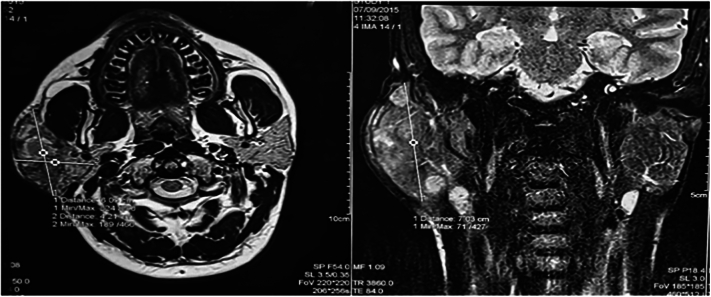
MRI showing axial and coronal reconstructions of a parotid mass exhibiting hypointensity on T1 and hyperintensity on T2.
